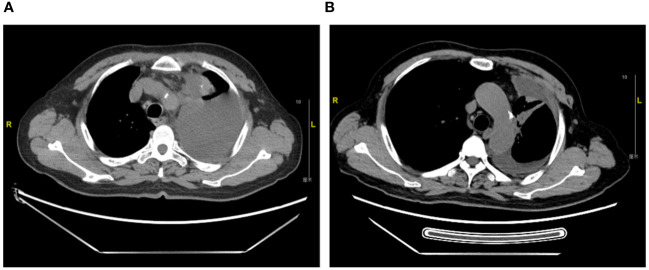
Thoracic computed tomography scans of Case 2. (A). Diagnosis of metastatic NSCLC in March 2022 (B). Partial response with a significant decrease in size of left upper lobe lung mass at 6-month follow up in September 2022.
